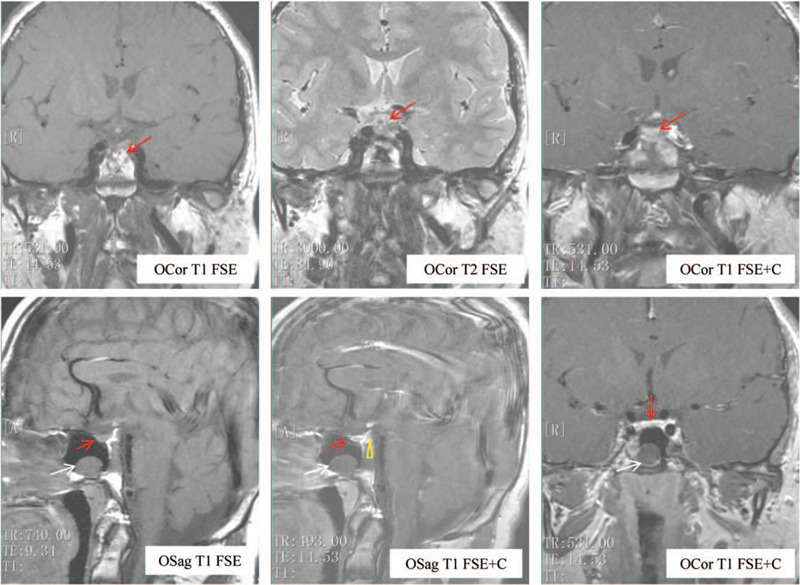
Improved findings on pituitary magnetic resonance imaging (MRI): The thickness of the anterior pituitary (red arrow) was about 3 mm, and a high signal in the saddle region was not observed on T1-weighted imaging. In the posterior lobe of the pituitary (yellow triangle), a high signal shadow was found in the lower margin of the hypothalamus, and enhancement was evident. The pituitary stalk was interrupted. There was no abnormal signal shadow in and around the saddle region. The optic chiasm was not compressed, and the bilateral cavernous sinus had a clear structure. However, the posterior fossa was small and a sphenoid sinus cyst was observed (white arrow). The MRI scan series was depicted below each image.
